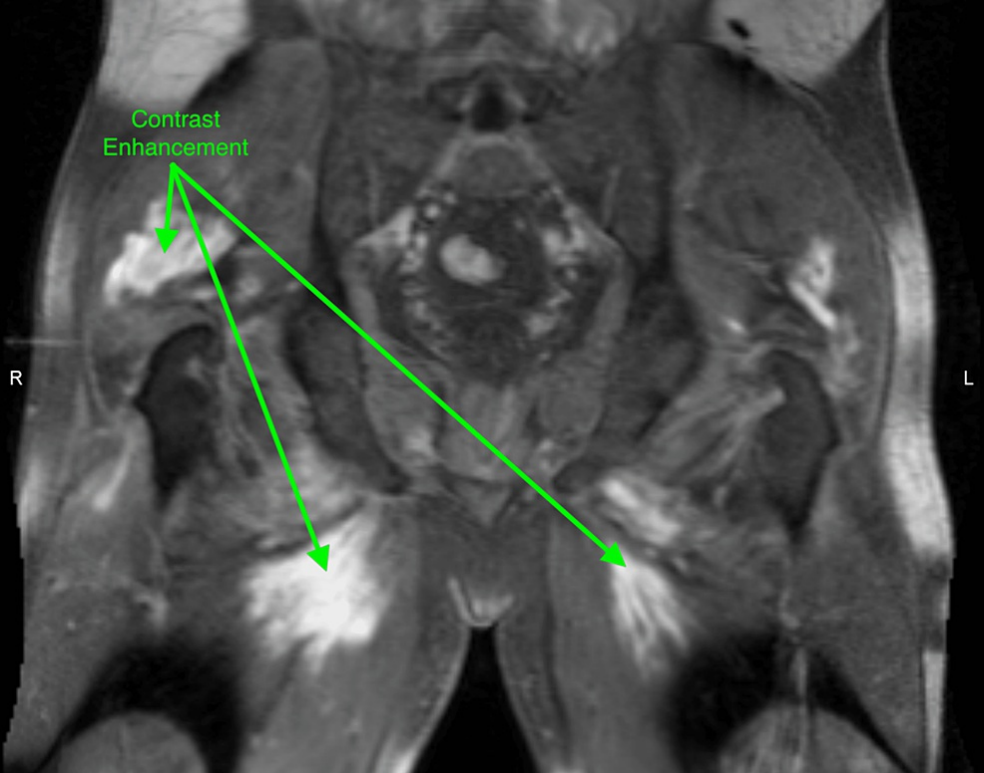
MRI of the pelvis with contrast - coronal VIBE MRI: magnetic resonance imaging; VIBE: volumetric interpolated breath-hold examination
